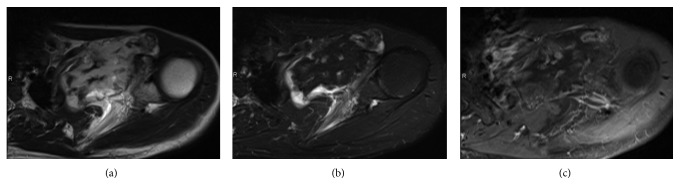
(a) T1-weighted magnetic resonance image (MRI). (b) T2-weighted MRI of fat suppression. (c) Contrast-enhanced T1-weighted preoperative MRI of fat suppression, axial view, showing a well-demarcated osseous lesion in the retroclavicular space with a thickened cartilaginous cap at the periphery of the tumor.
